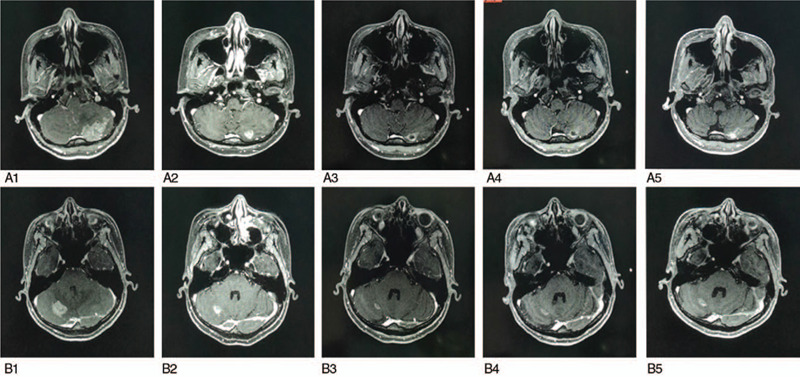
Evaluation of multiple metastases in both sides of cerebellum by MRI in 12-month follow-up. (A1–A5) Evaluation of metastatic lesion in left cerebellar hemisphere; (B1–B5) evaluation of metastatic lesion in right cerebellar hemisphere.
